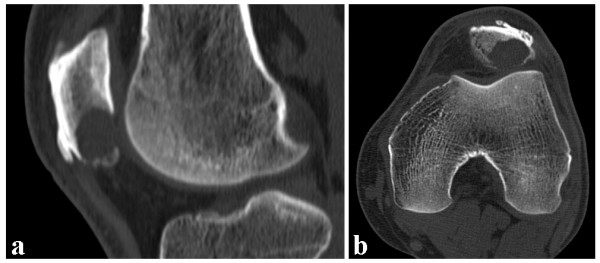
Computed tomography of the left knee. CT scan of the left patella showing an osteolytic lesion within the inferior pole of the patella. Note the cortical thinning/breakthrough as a sign for the aggressiveness of the lesion. (a) sagittal, (b) axial sequence.
